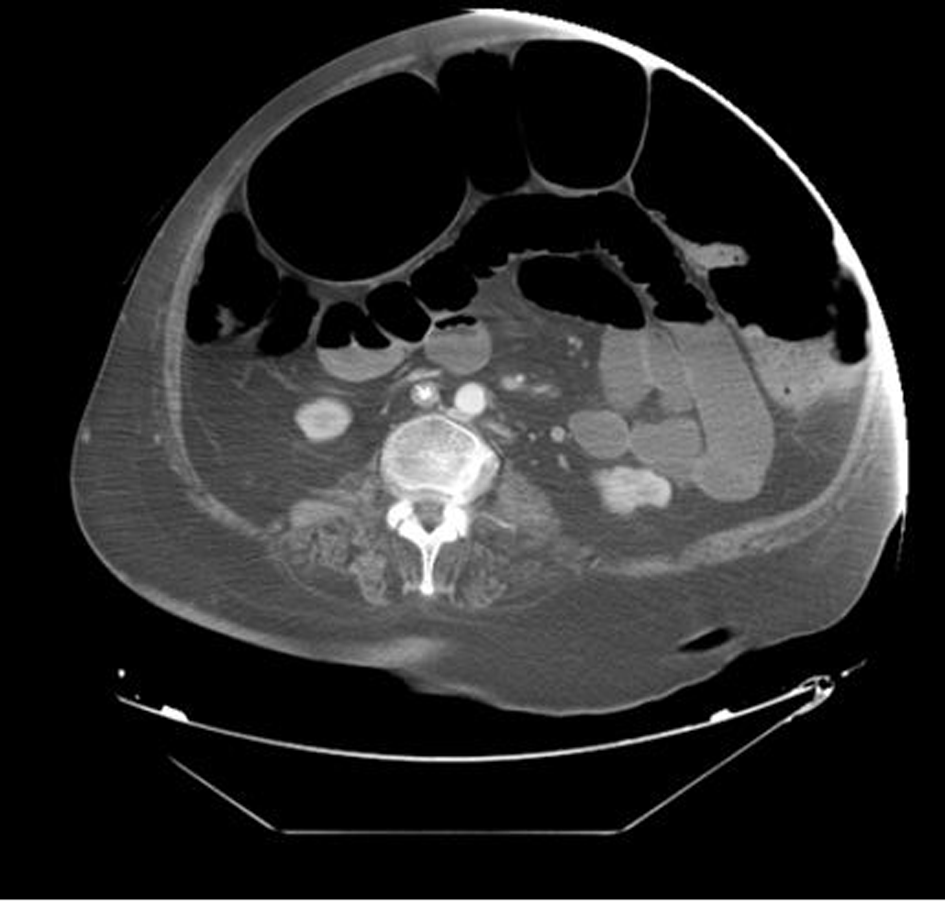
Spiral CT-Abdomen, transverse section demonstrating massive colonic dilatation.
